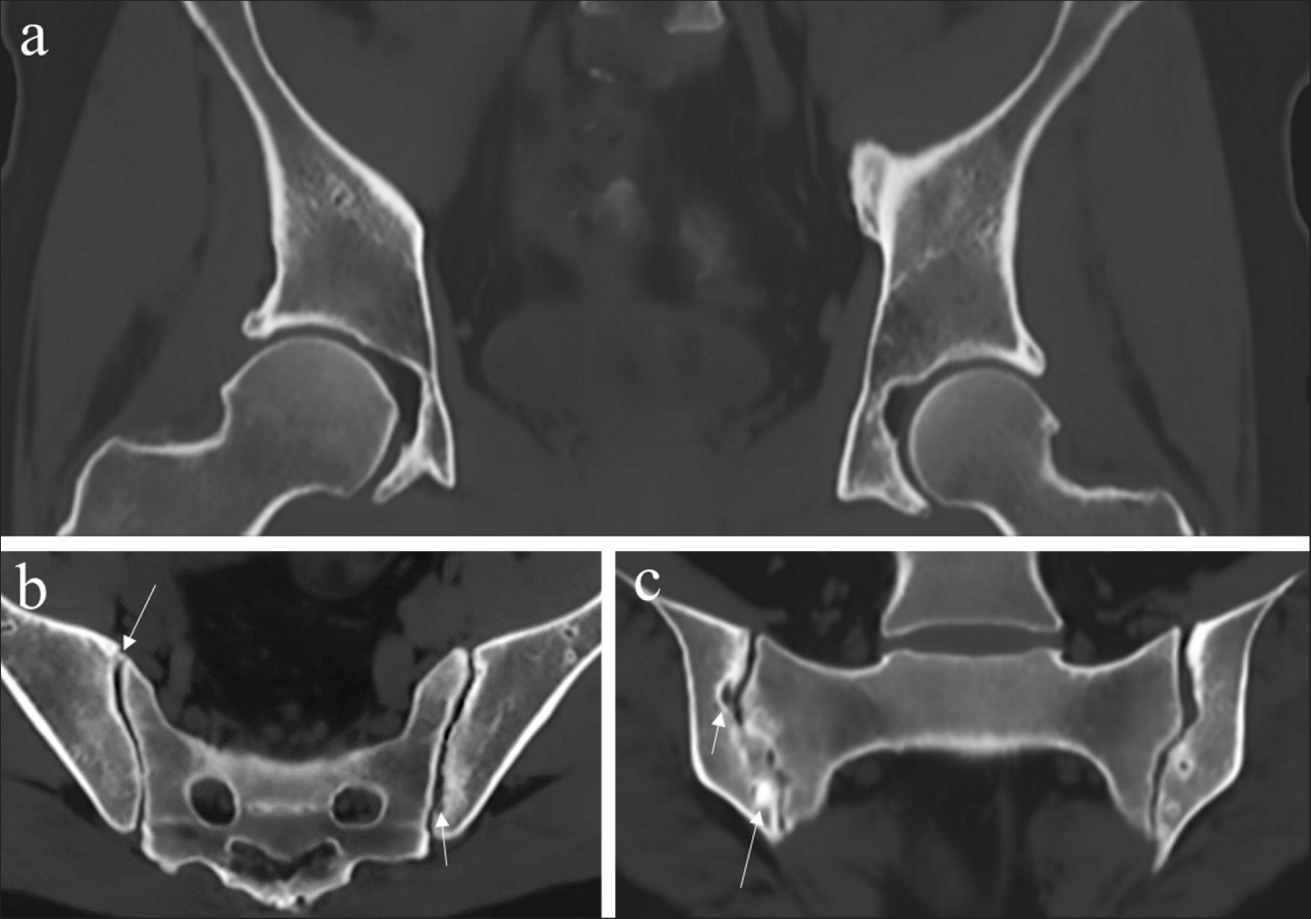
MRI-based synthetic CT. (a) Coronal image with bilateral degenerative hip disease with subchondral sclerosis and osteophytes in a 47-year-old male. (b) Axial image of erosions (short arrow) with subchondral sclerosis in the left sacroiliac joint and incipient bony bridging in the right sacroiliac joint (long arrow) in a 30-year-old male with sacroiliitis. (c) Semicoronal image of the sacroiliac joint shows erosions (short arrow) with subchondral sclerosis (long arrow) in the right sacroiliac joint in a 42-year-old female with sacroiliitis.
